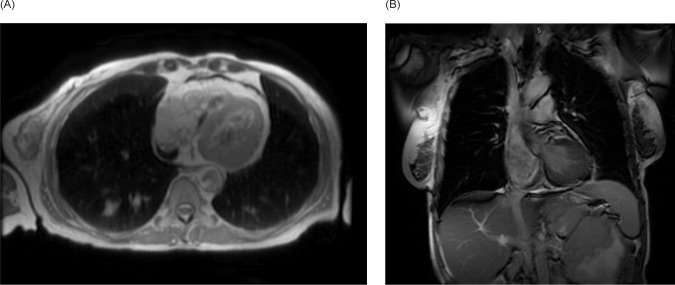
Cardiac MRI. (A) Transverse and (B) coronal views demonstrating a large intra-cardiac tumor occupying the majority of the right atrium extending through the myocardium into the epicardial space and through the tricuspid valve into the right ventricle.
